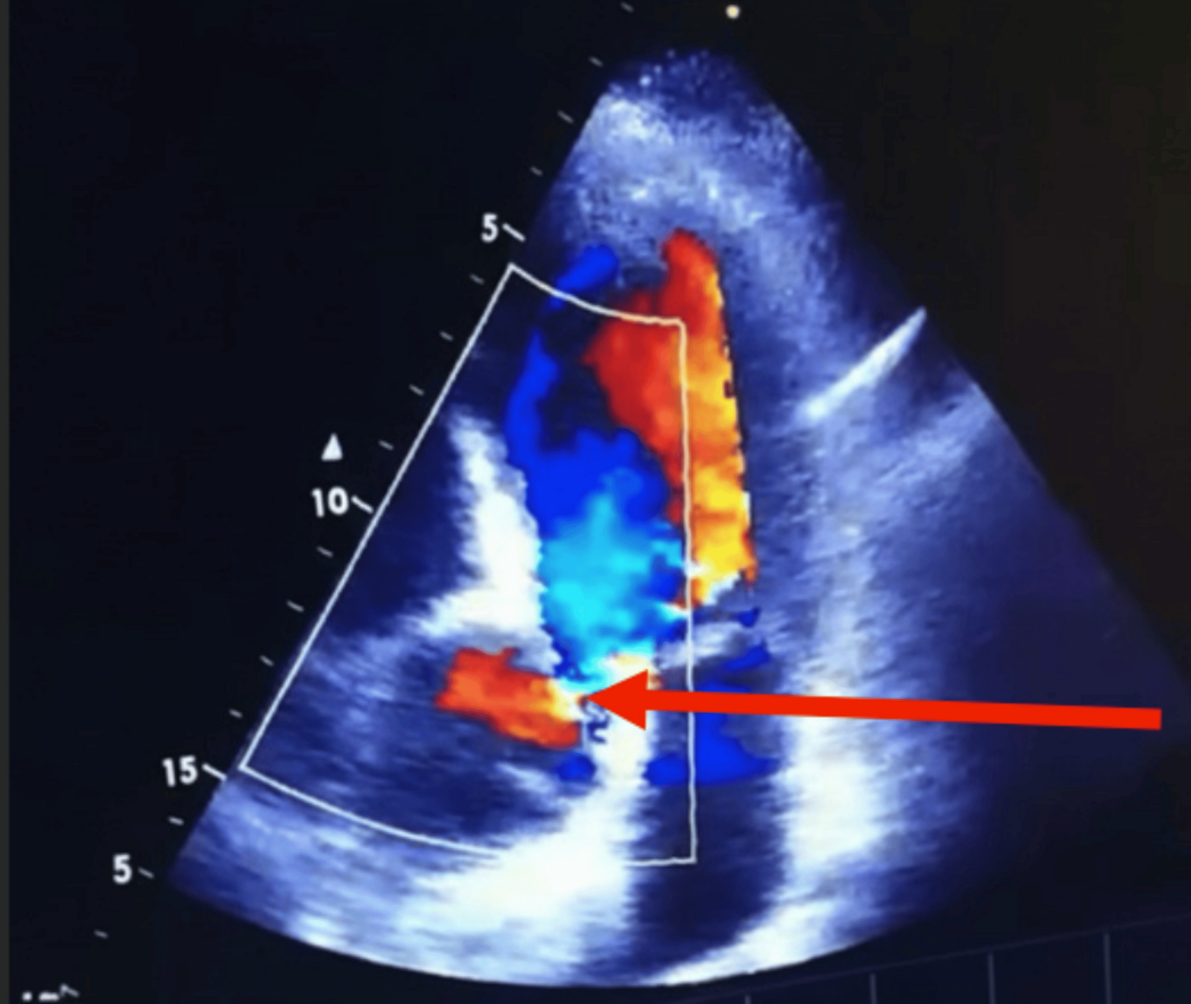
Two-dimensional apical four-chamber view on transthoracic echocardiography with Doppler demonstrating aortic regurgitation (arrow) The red arrow indicates the aortic valve, and the blue mosaic jet represents retrograde flow from the aorta into the left ventricle during systole, consistent with aortic regurgitation.
